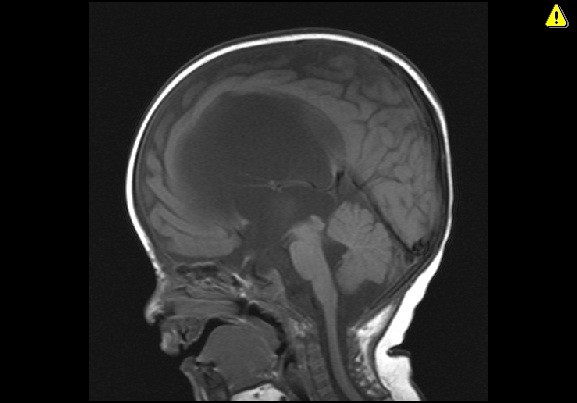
Sagittal T1-weighted magnetic resonance image four weeks after the endoscopic third ventriculostomy. Demonstrates unchanged ventricular size despite patent ventriculostomy
